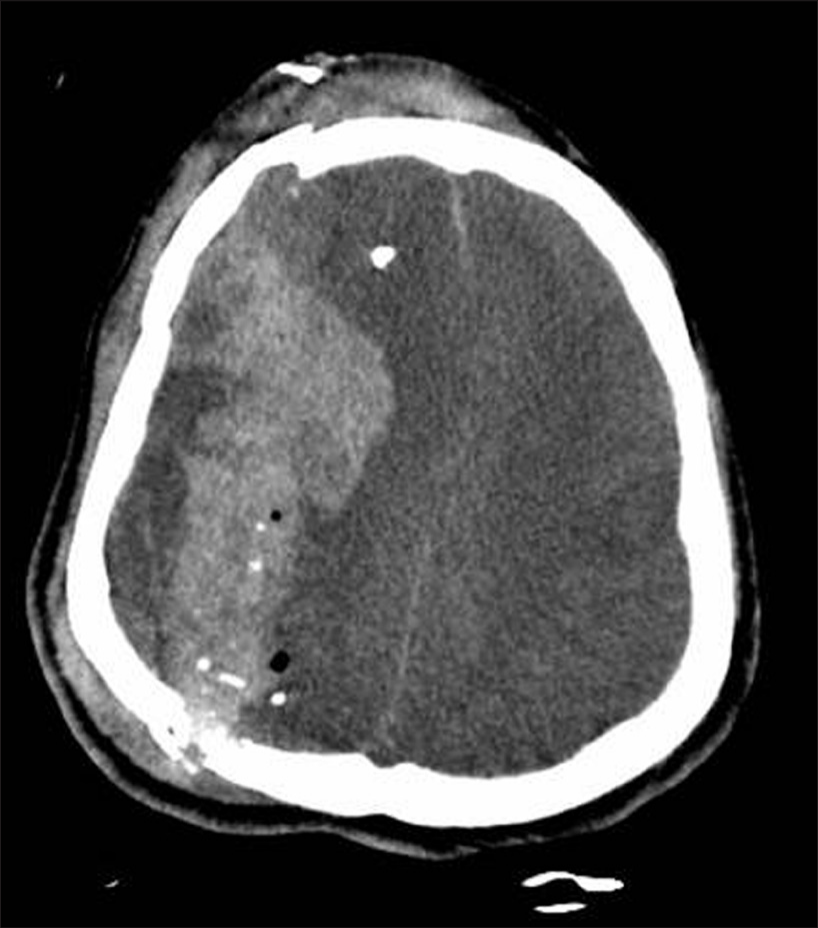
CT image of patients skull fractures and fragmentation with acute intracerebral hemorrhage and midline shift
